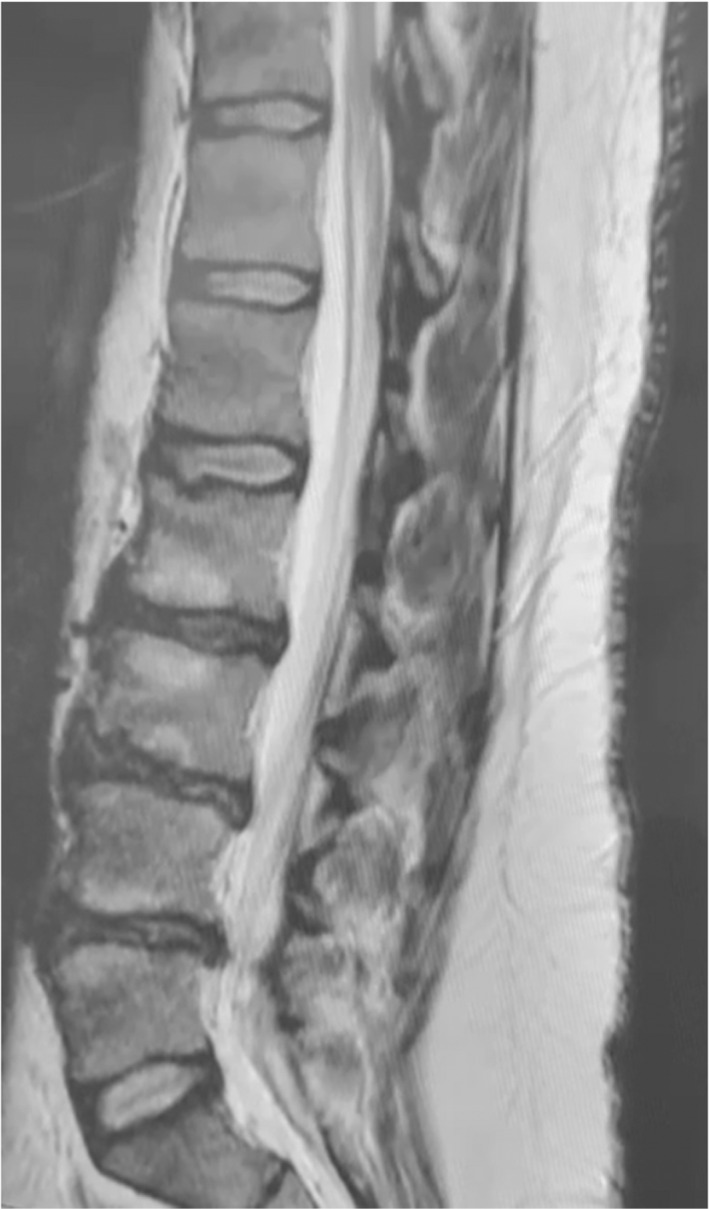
MRI of the lumbar spine before treatment showing bulging of the discs between L5 and L2 region
